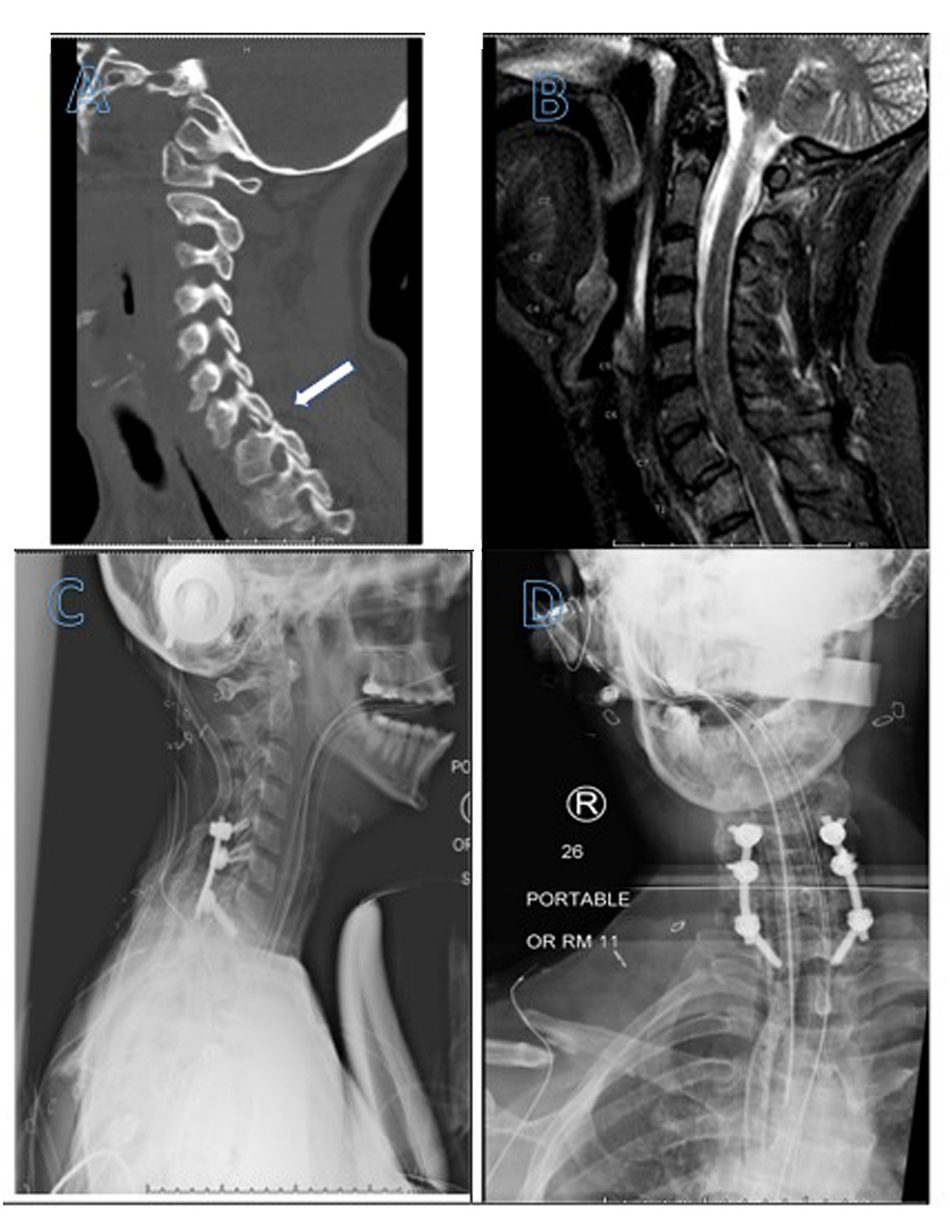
Neuroimaging findings of Case #2 A-B: Sagittal view computed tomography image and MRI of the cervical spine revealing a fracture-dislocation at C7-T1 with perched facets and 2-3 mm of anterolisthesis of C7 on T1, C-D: Anterior and lateral radiographs demonstrating postoperative reduction of the C7-T1 fracture and C5-T1 posterior instrumented fusion construct
